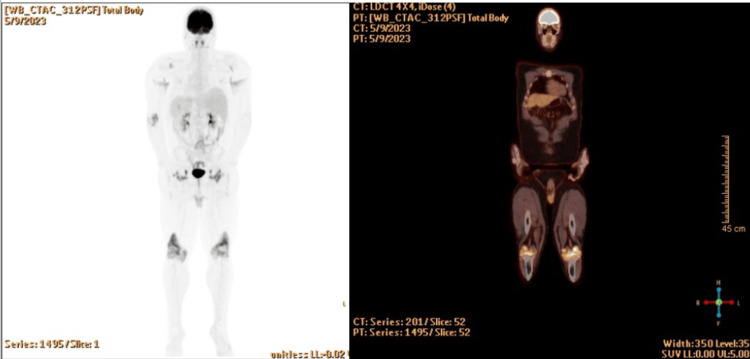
A repeat PET/CT scan shows hypermetabolism involving multiple joint spaces with associated bone erosion, as well as hypermetabolic cutaneous thickening in axillary and perineal regions
